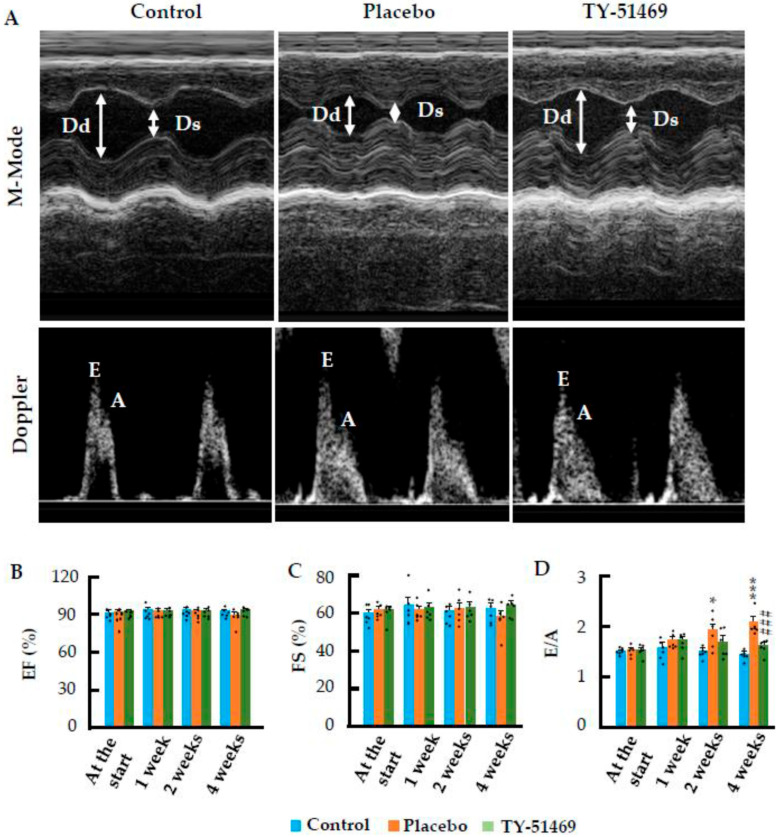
Effects of chronic Ang II infusion on left ventricular ejection fraction (LVEF), fractional shortening (FS), and the ratio of early (E) to late (A) ventricular filling velocities (E/A). (A): Representative M-mode echocardiograms and Doppler spectra of mitral inflow in the control group and Ang II-infused hamsters treated with placebo or TY-51469. (B–D): Changes in LVEF, FS, and the E/A ratio in the control group and in Ang II-infused hamsters treated with placebo or TY-51469. Dd, LV end-diastolic dimension; Ds, LV end-systolic dimension; E, early diastolic inflow velocity; A, late diastolic inflow velocity. * *p* < 0.05, * *p* < 0.001 vs. control; ### *p* < 0.001 vs. placebo.
